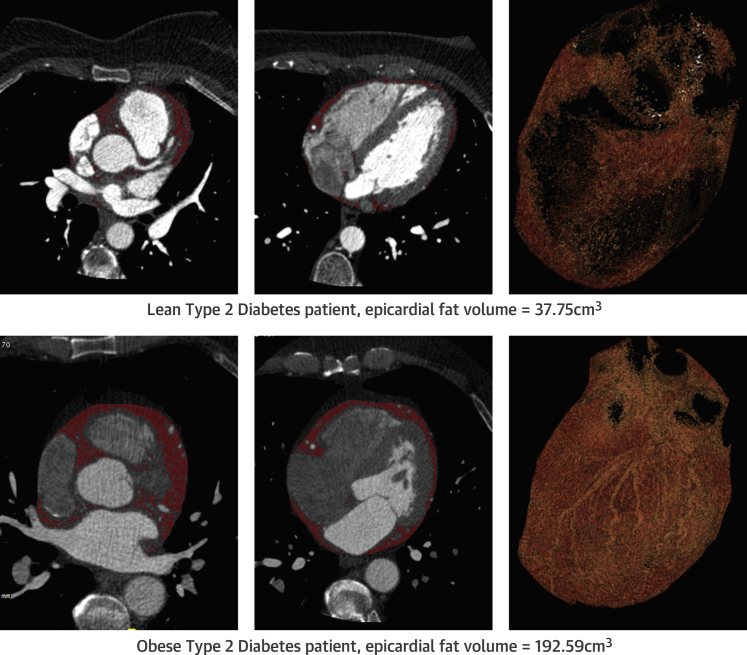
Representative Examples of CT Epicardial Fat Volumes in a Lean and an Obese Patient With T2D (Top) Lean patient with T2D with epicardial fat volume 37.75 cm^3^. (Bottom) Obese patient with T2D with epicardial fat volume 192.59 cm^3^. Abbreviations as in Figure 1.
